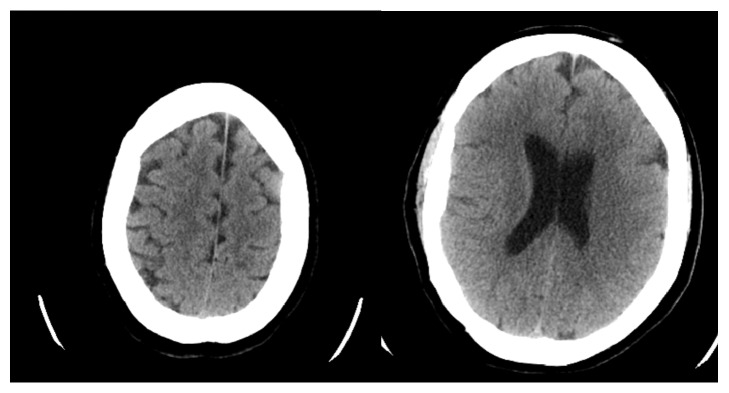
Brain CT on presentation.
